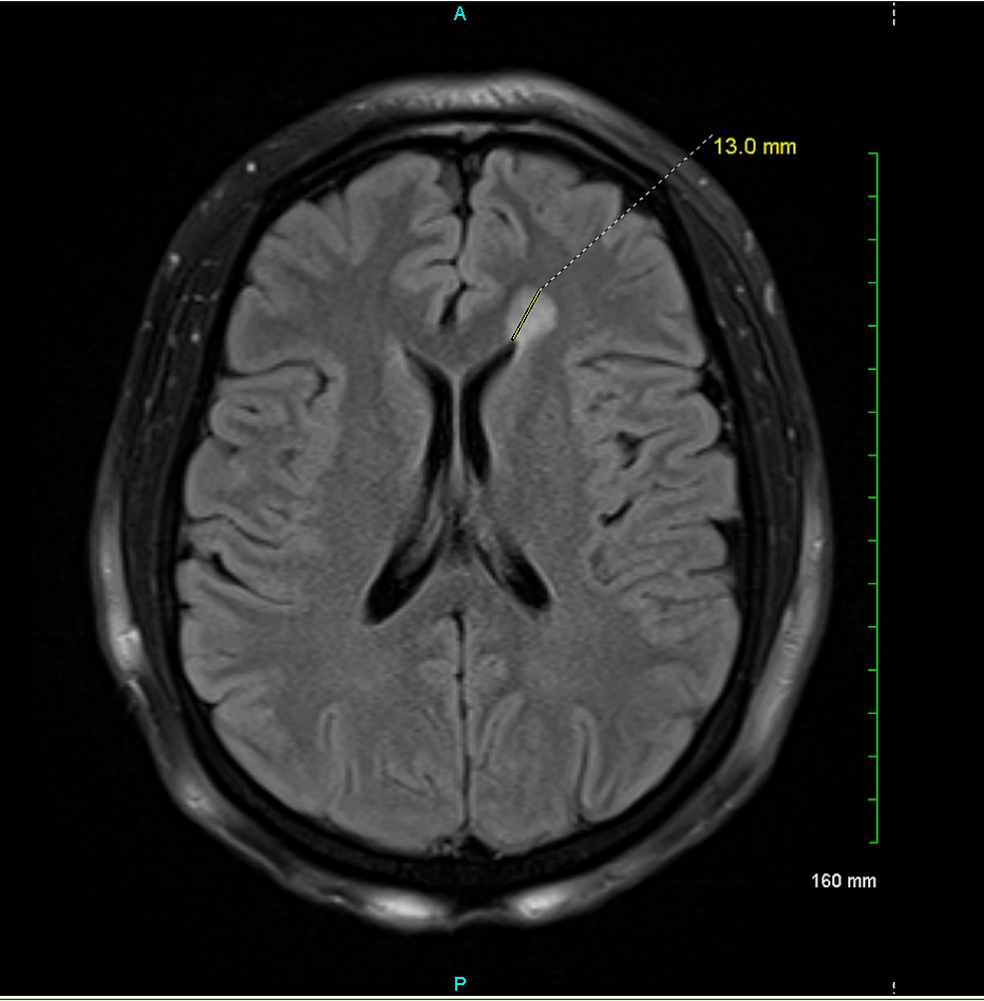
A round 13 mm T2 FLAIR signal hyperintense lesion in the left frontal white matter abutting the frontal horn of the left lateral ventricle. This lesion does not have restricted diffusion and lacks contrast enhancement. FLAIR = fluid-attenuated inversion recovery
